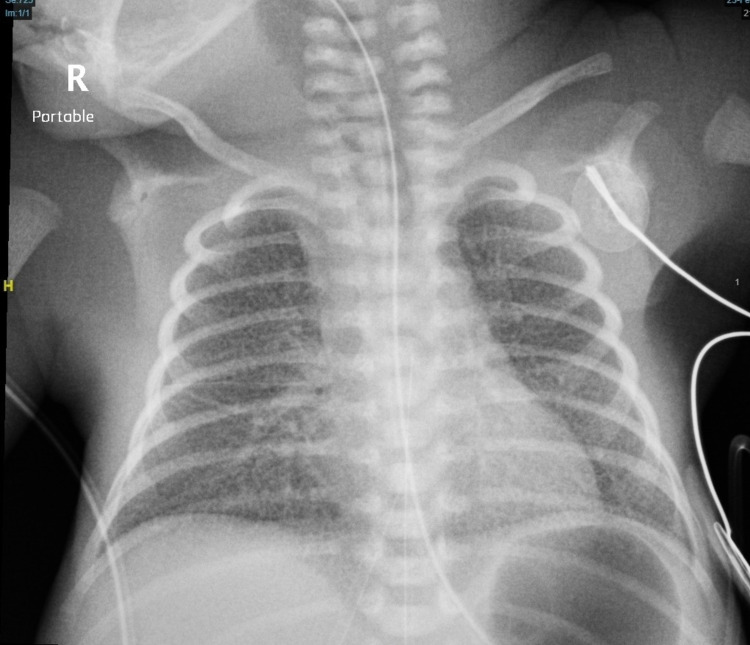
Chest radiography revealed better expansion of both lung fields compared with a previous study. However, bilateral diffuse granular infiltrate was noted.
